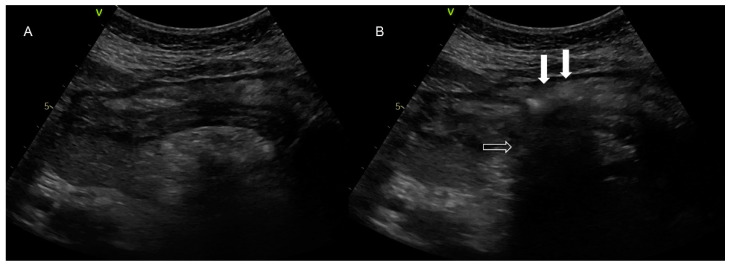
Abdominal ultrasonography. In picture (A), there is a presentation of the stomach at the center of the image in the longitudinal axis, while the hyperechoic structure beneath is pancreatic tissue. In picture (B), the image is obtained after instillation of a “flash” of air (white arrows) through the nasogastric tube. The pancreatic tissue is obscured (empty arrows), as an amount of air interferes between the ultrasonographic beam and the pancreas.
